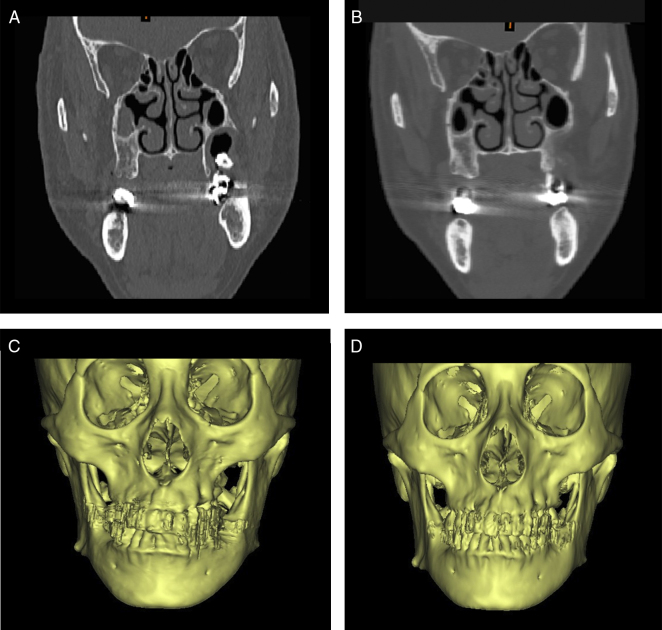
CT and 3D reconstruction images of bone removal method. (A and C) Before operation. (B and D) Images postoperatively shows normal shape.
